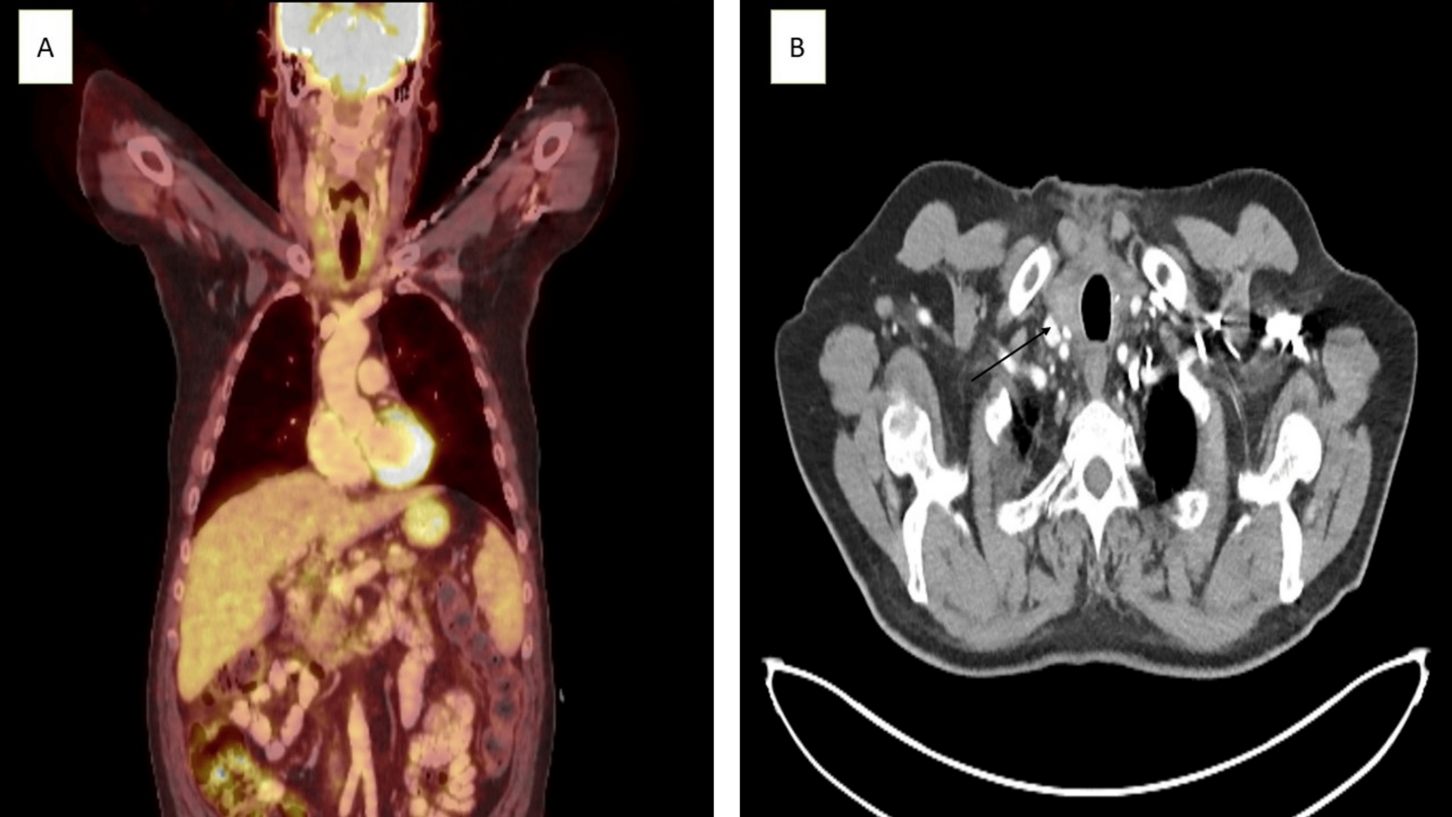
A. Six weeks post operative PET-CT showing no pathological uptake in the thyroid gland, neck lymph nodes, chest or abdomen. B. Post operative axial CT showing the thyroid gland remnants (black arrow).
